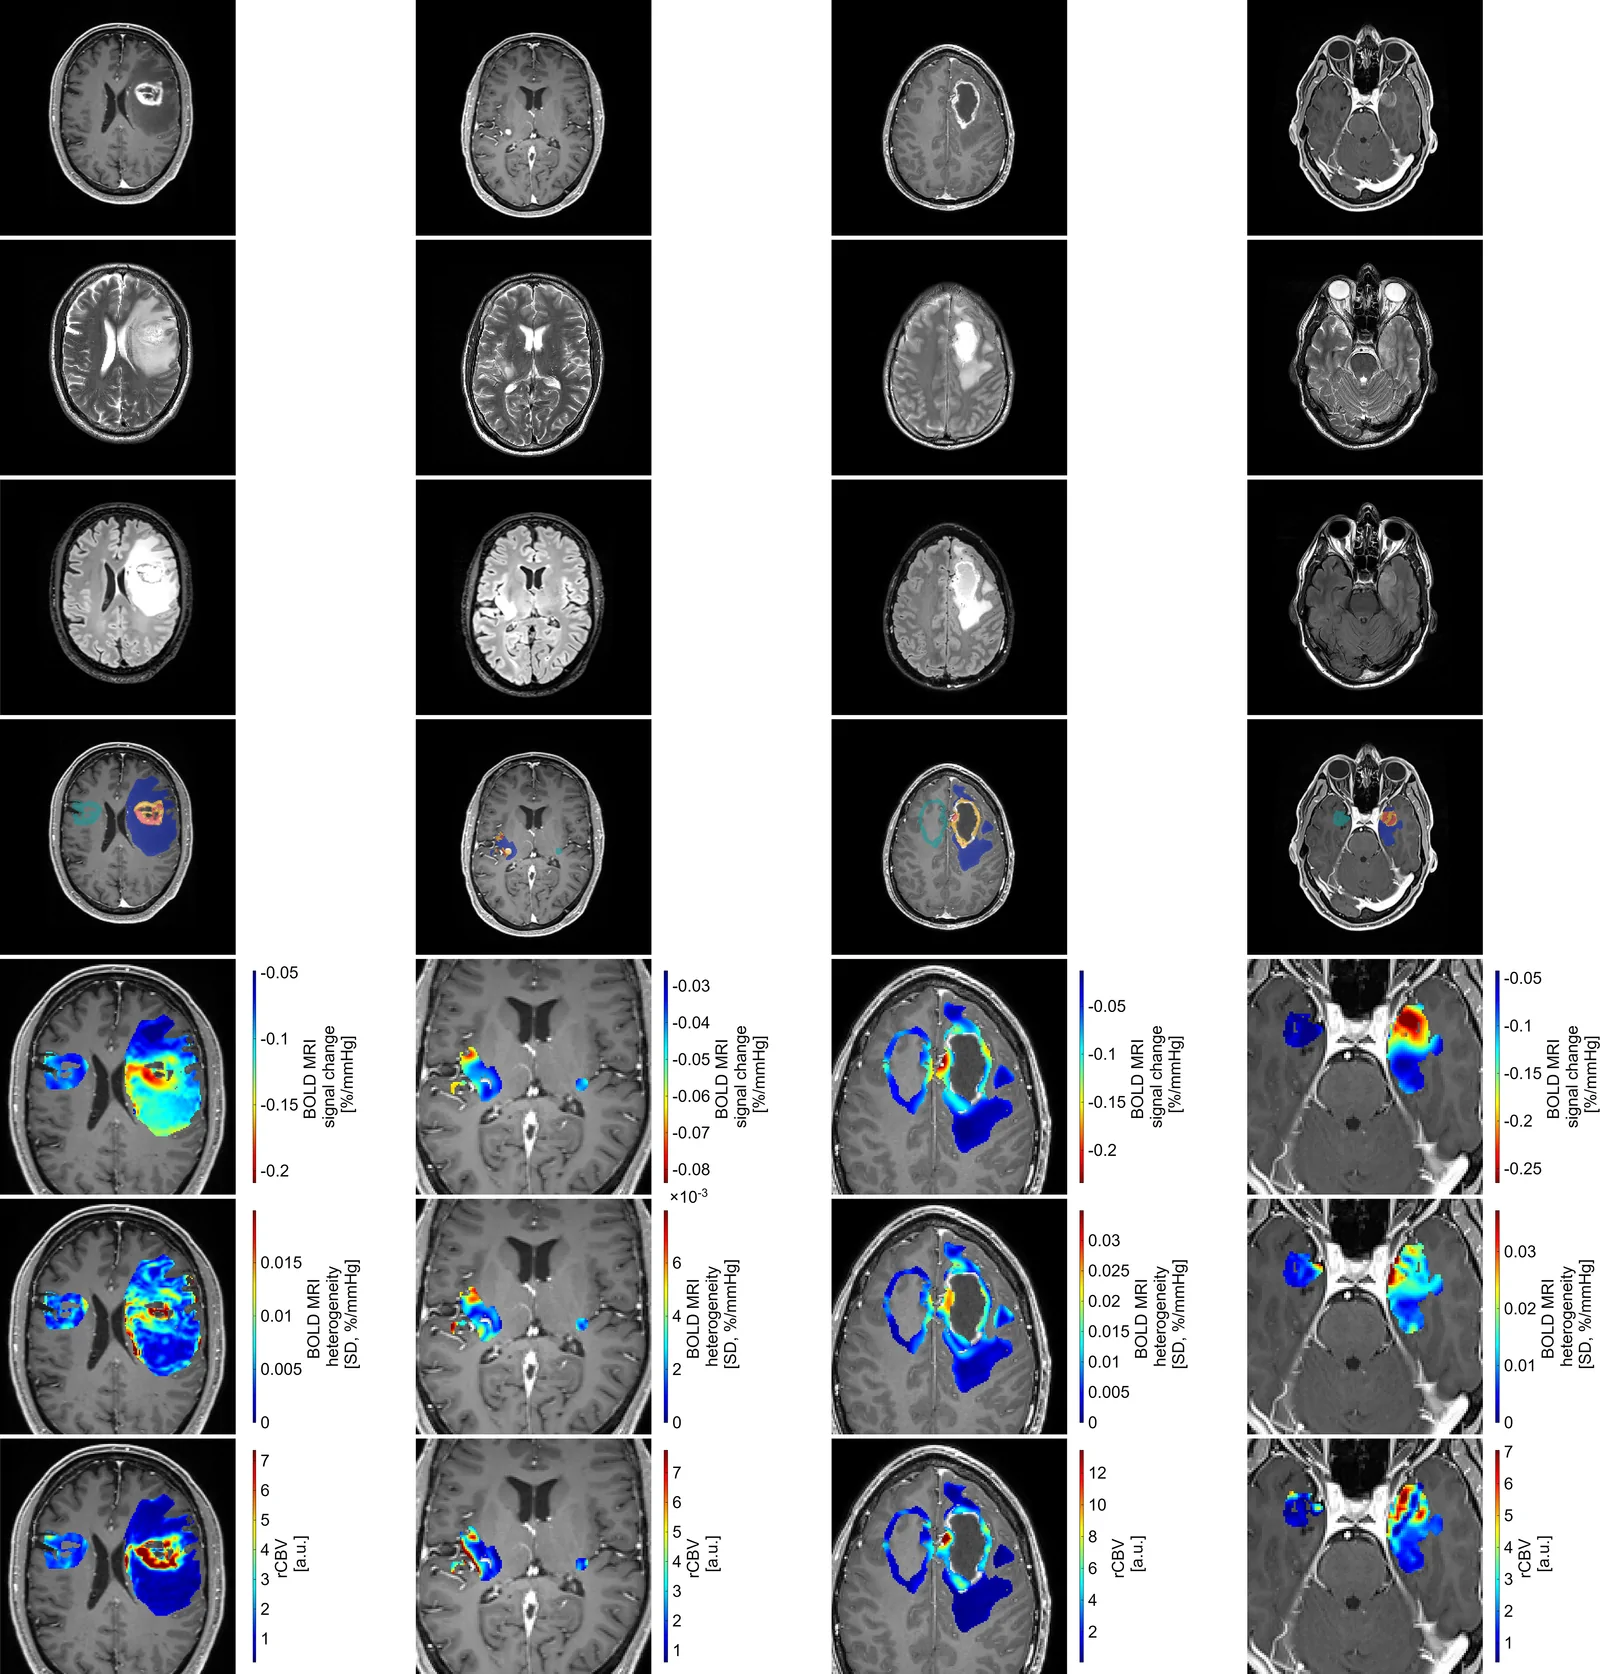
MRI data of four patients with glioblastoma. First row: T1-weighted post-contrast; second row: T2-weighted; third row: fluid-attenuated inversion recovery (FLAIR); fourth row: depiction of the volumes of interest. Red = high angiogenic tumor, orange = low angiogenic tumor, blue = edema, green = mirrored contrast-enhancing tissue; fifth row: BOLD signal change; sixth row: BOLD signal heterogeneity; seventh row: relative cerebral blood volume (rCBV)
